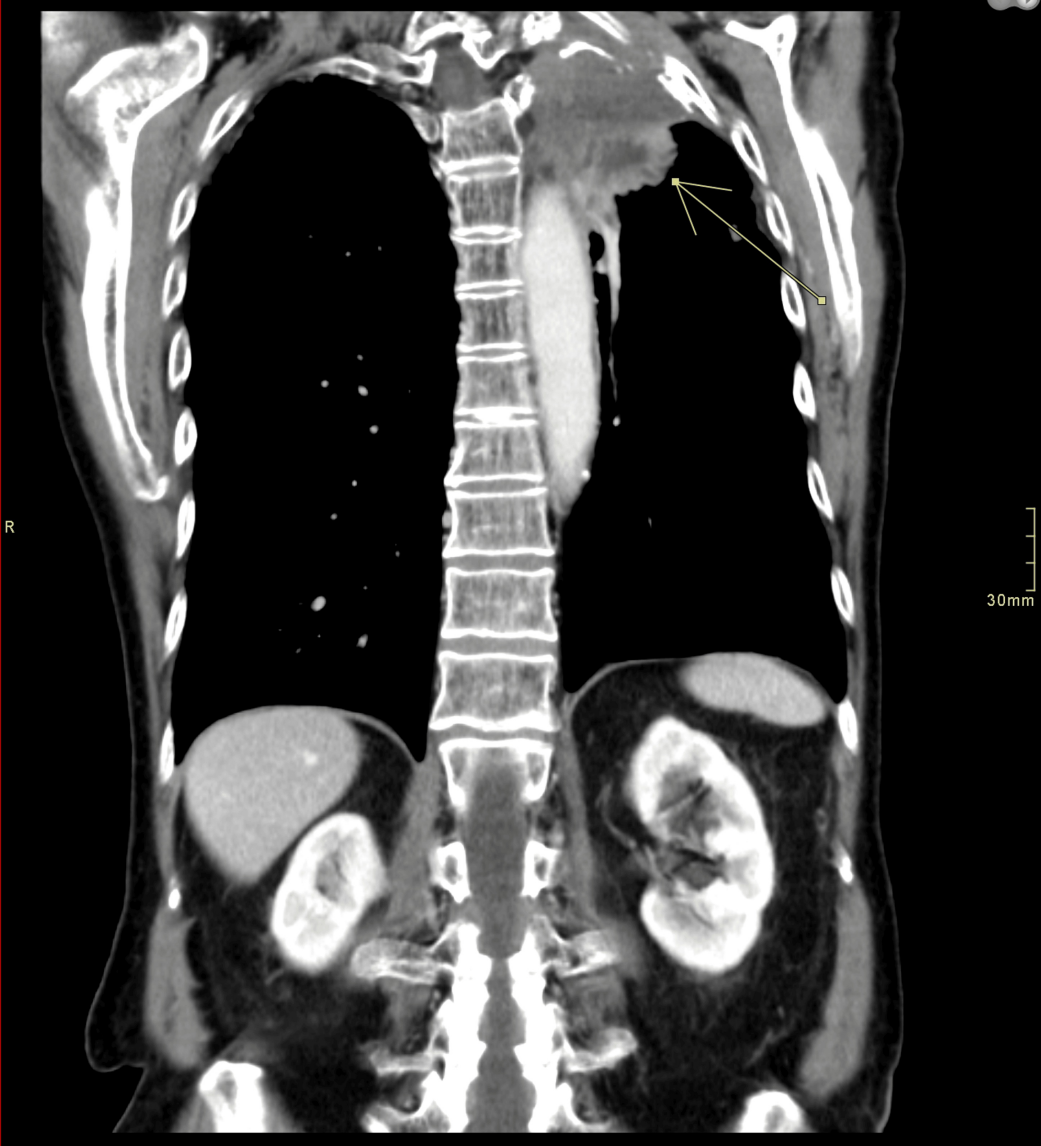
Coronal CT thorax and abdomen/mediastinal window with IV contrast revealing a new mass in the LUL suggesting recurrence of the Pancoast tumor LUL: left upper lobe The arrow points to the lung lesion
